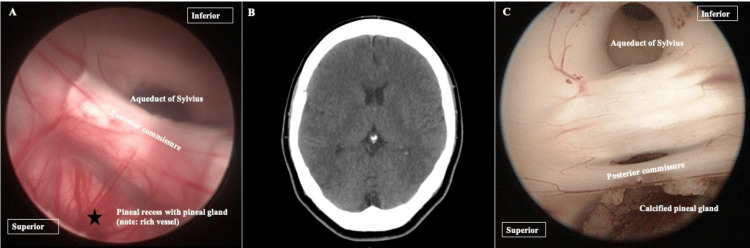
The pineal gland. (A) The neuroendoscopic view shows the pineal recess with a rich network of blood vessels at the area of the pineal gland (star). (B) In a noncontrast CT brain, when there is a presence of calcium, the pineal gland will appear hyperdense (circle). (C) It is uncommon to see the actual features of a calcified pineal gland.
